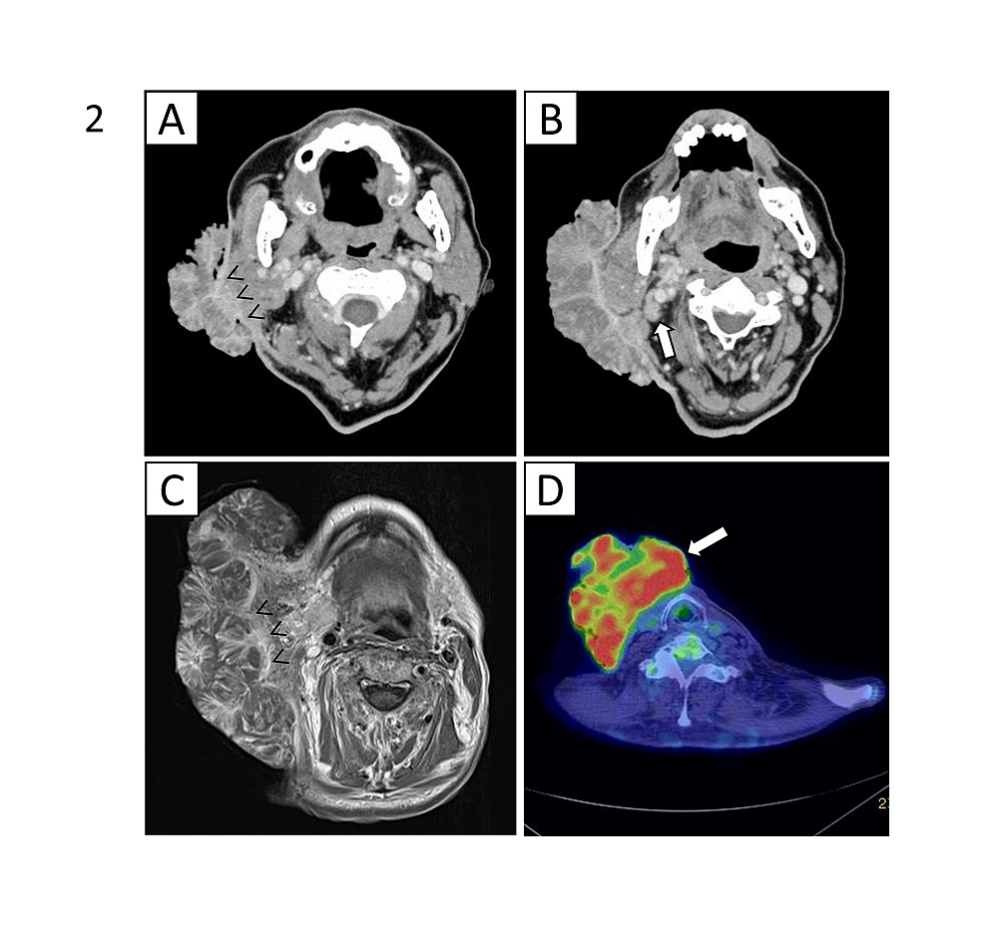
Representative images of contrast-enhanced computed tomography (A, B), T1-weighted gadolinium-enhanced magnetic resonance imaging (C), and positron emission tomography/computed tomography (D). (A) The tumor appeared to invade the parotid gland (arrow head). (B) Enlarged lymph nodes beneath the tumor (arrow). (C) The tumor appeared to invade the sternocleidomastoid muscle (arrow head). (D) A high accumulation (maximum standardized uptake value of 8.88) of 18F-fluoro-2-deoxyglucose was observed in the cutaneous tumor (arrow).
